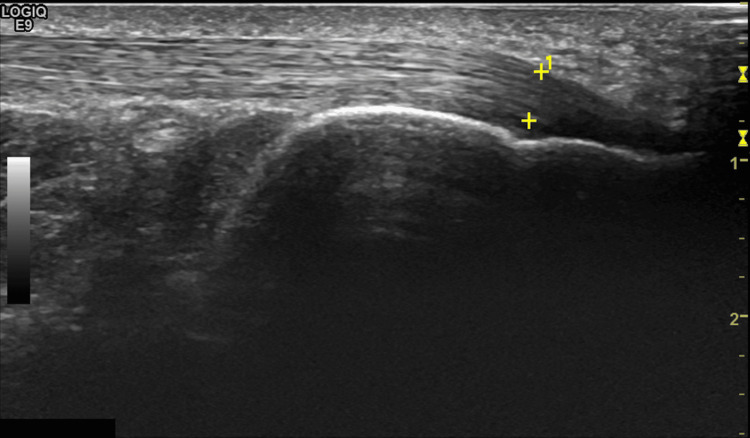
Ultrasound imaging: longitudinal image of Achilles tendon showing a normal fibrillar pattern
